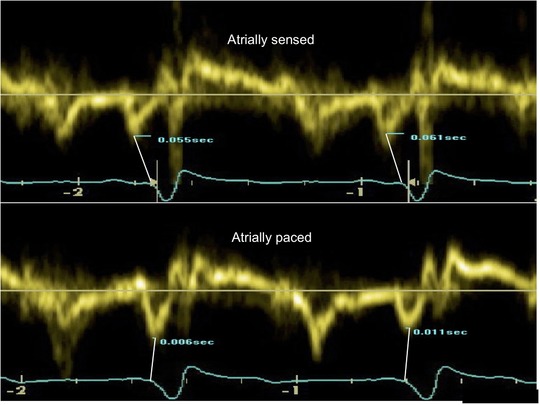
Example measurement of change in the timing of left atrial contraction when changing from a sensed to a paced atrium, at the same programmed AV delay. Tissue Doppler imaging across the septal wall of the mitral valve annulus, during sensed and paced atrial modes of biventricular pacing. The same AV delay was programmed for both modes. The time of contraction of the left atrium (peak of A’) was measured from a fixed time point (the onset of the QRS) in order to allow precise measurements of alterations in the time of left atrial contraction as a result of pacing changes. In this example, the A’ wave can be seen to be shifted to the right when atrially paced and this change denotes delay of left atrial contraction during atrial pacing. AV = atrioventricular [Color figure can be viewed at http://wileyonlinelibrary.com]
